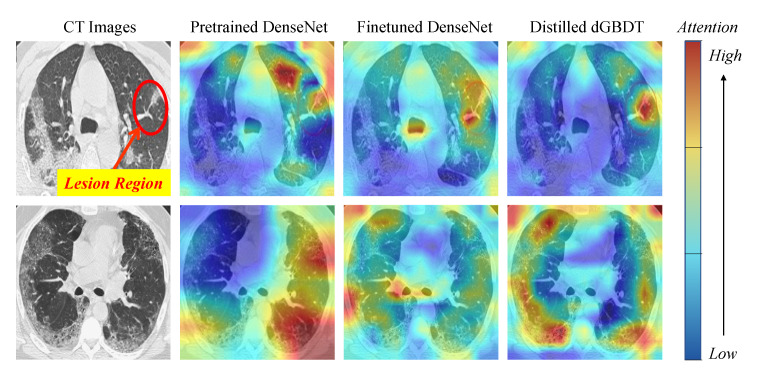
CAM visualizations for the ImageNet pre-trained DenseNet, the COVID-19 CT fine-tuned DenseNet, and the distilled dGBDT. The first row is an instance for a COVID-19 CT (in which the lesion region is labeled by a human doctor), while the second is for a Non-COVID-19 CT.
